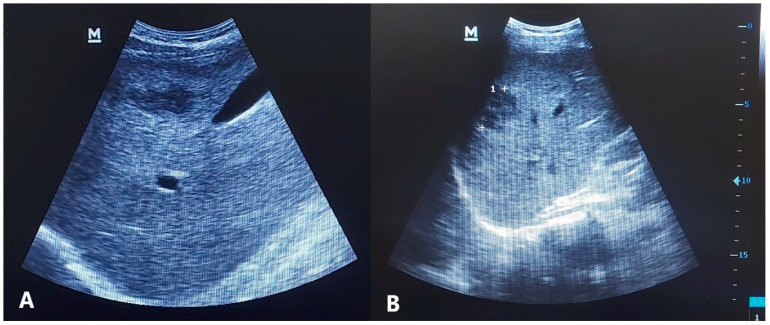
Pattern of different hypoechoic hepatic focal lesions by ultrasound in two patients (A,B) at enrollment in the study.
